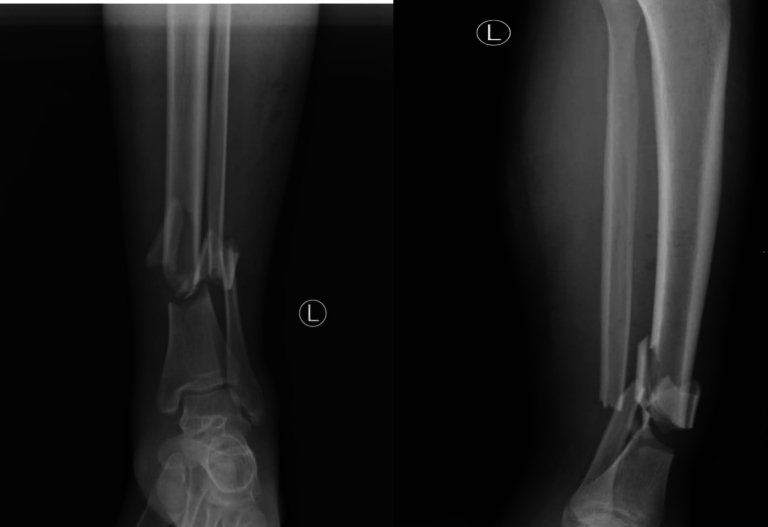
Preoperative anteroposterior and lateral radiographic imaging showing segmental fracture at the junction of the middle and distal third of his tibia.
